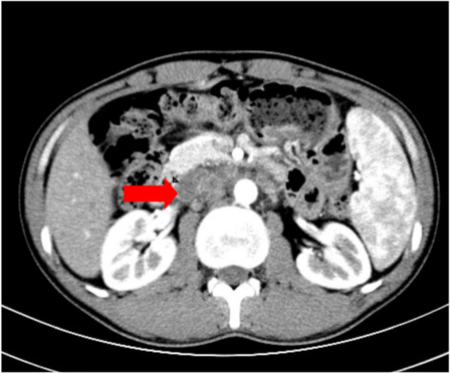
CT scan image (axial view) showing many swollen lymph nodes adjacent to the abdominal aorta in the retroperitoneal space but no discernible mass
